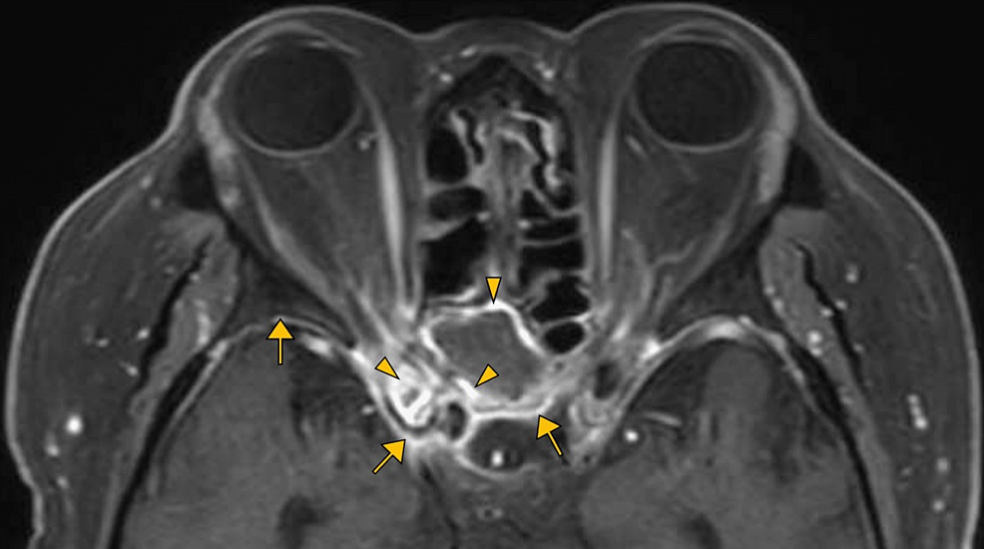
Contrast-enhanced magnetic resonance imaging (fat-suppressed T1-weighted image) shows mucosal thickening (arrowheads) and fluid retention in the right posterior ethmoid sinus and spread of the contrast medium over the dura around the right posterior ethmoid sinus (arrows) and bilateral optic nerve tracts (right predominant)
